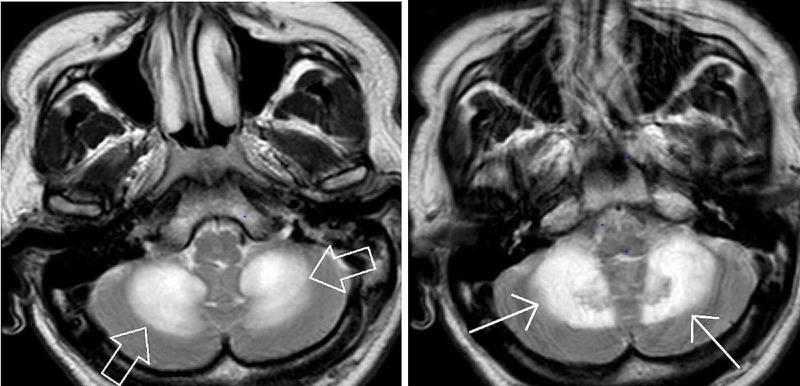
MRI brain without contrast
